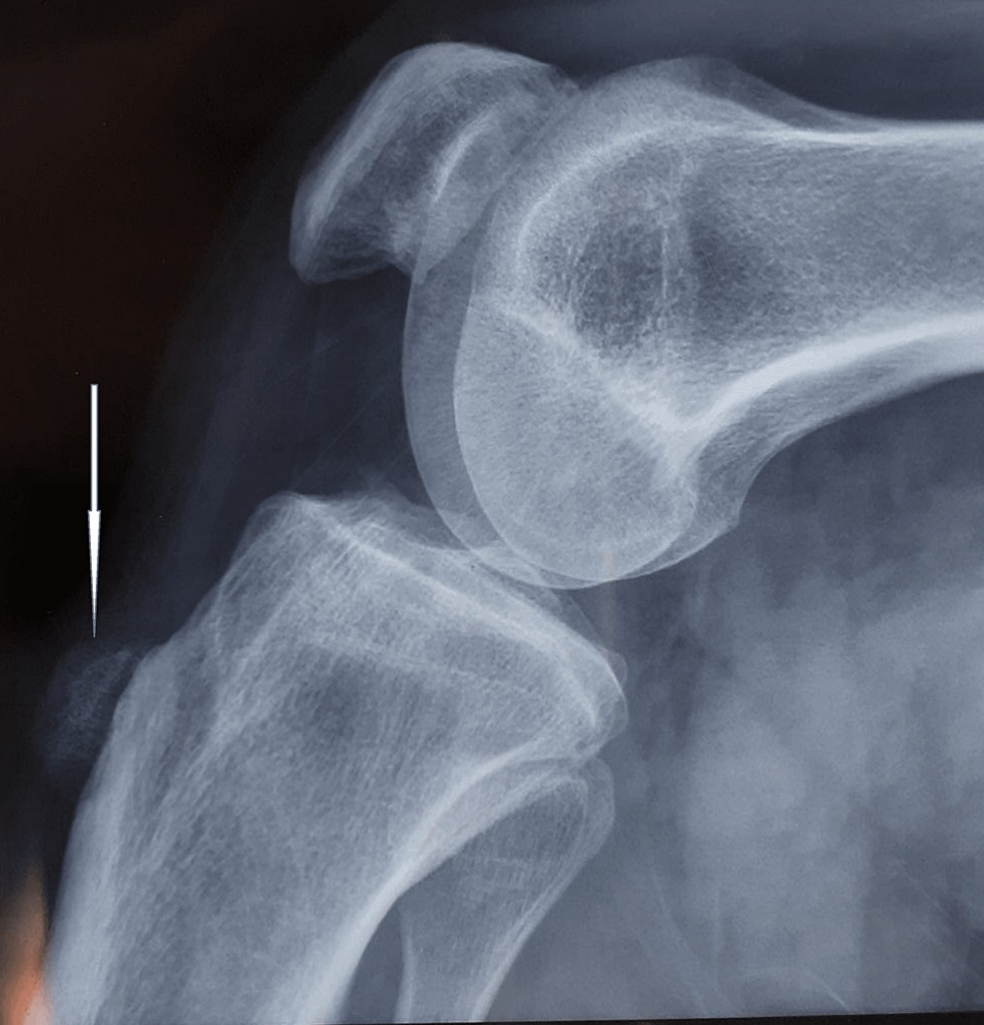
Lateral X-ray demonstrating ossification anterior to the tibial tuberosity
